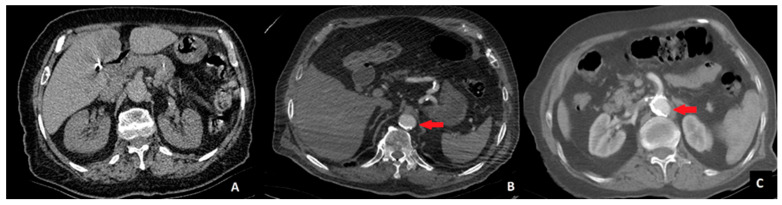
Computed tomography images of patients. A—no calcification (Stage 0), B—calcified area less than 50% of the aortic circumference (Stage 1), and C—calcified area more than 50% of the aortic circumference (Stage 2). The area of calcification is indicated by a red arrow.
